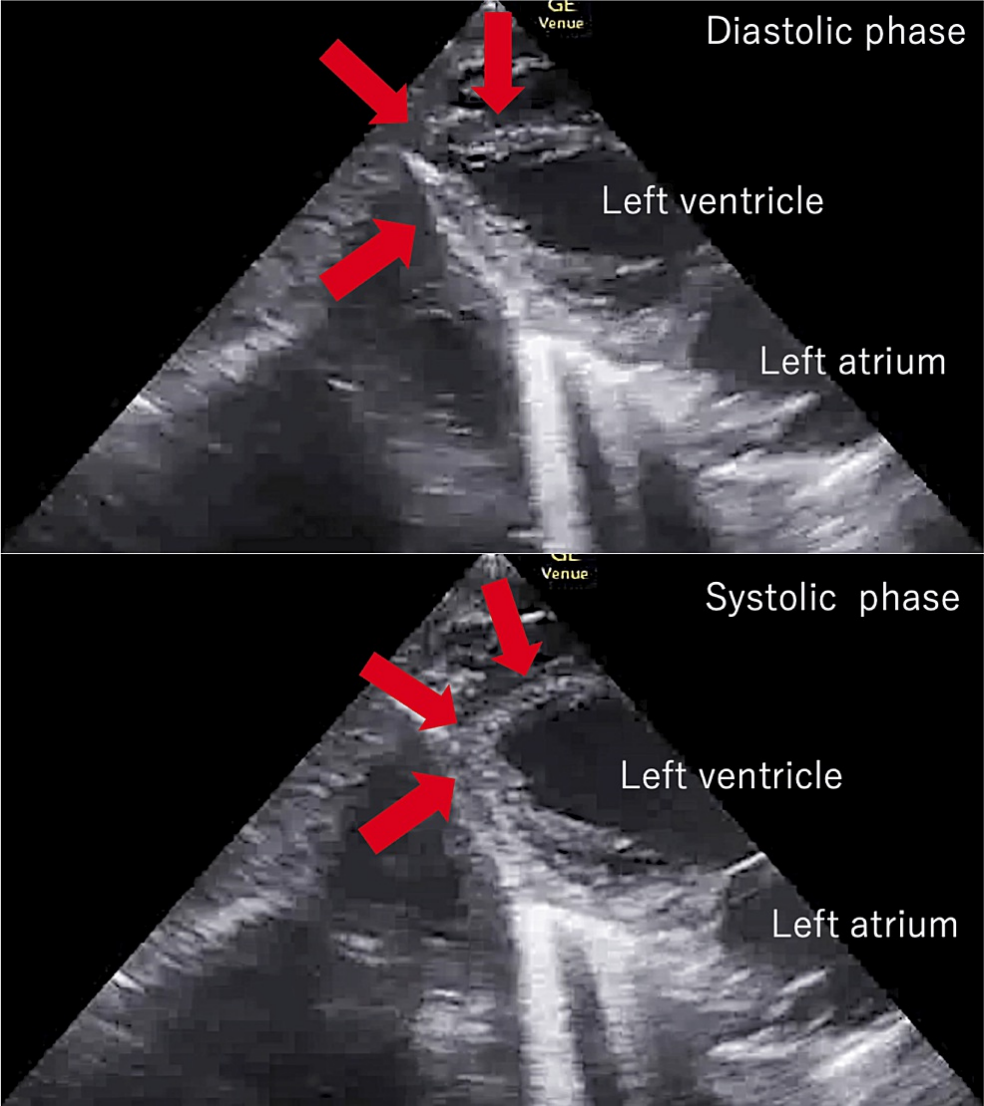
Echocardiogram on admission. Comparison of diastolic and systolic views. Only the apex of the left ventricle (red arrow) contracted.
